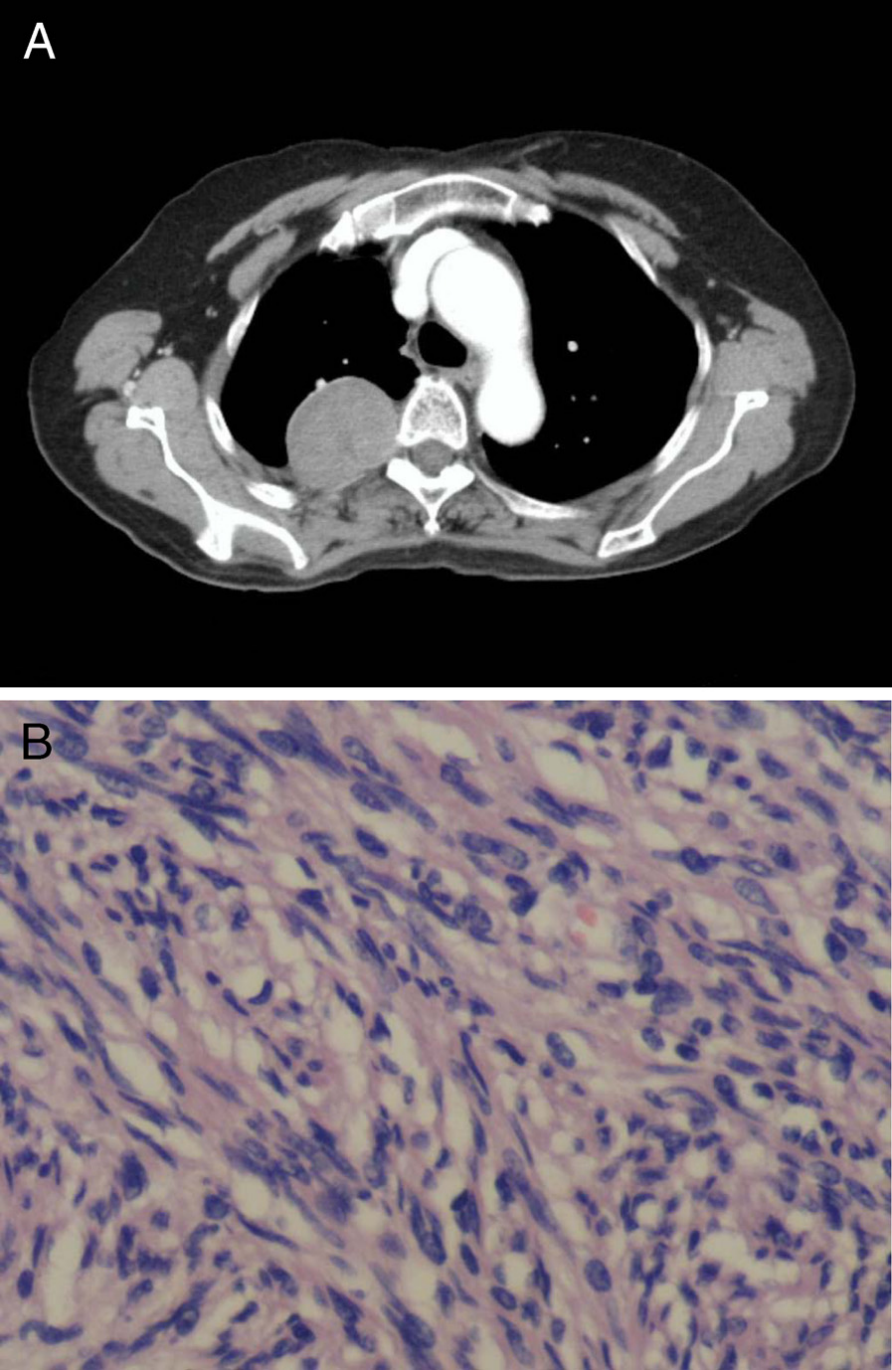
A - Chest CT scan showing a soft tissue density in the right upper lung. B - Pathological section showing bundles of spindle-shaped cells. HE × 400.
